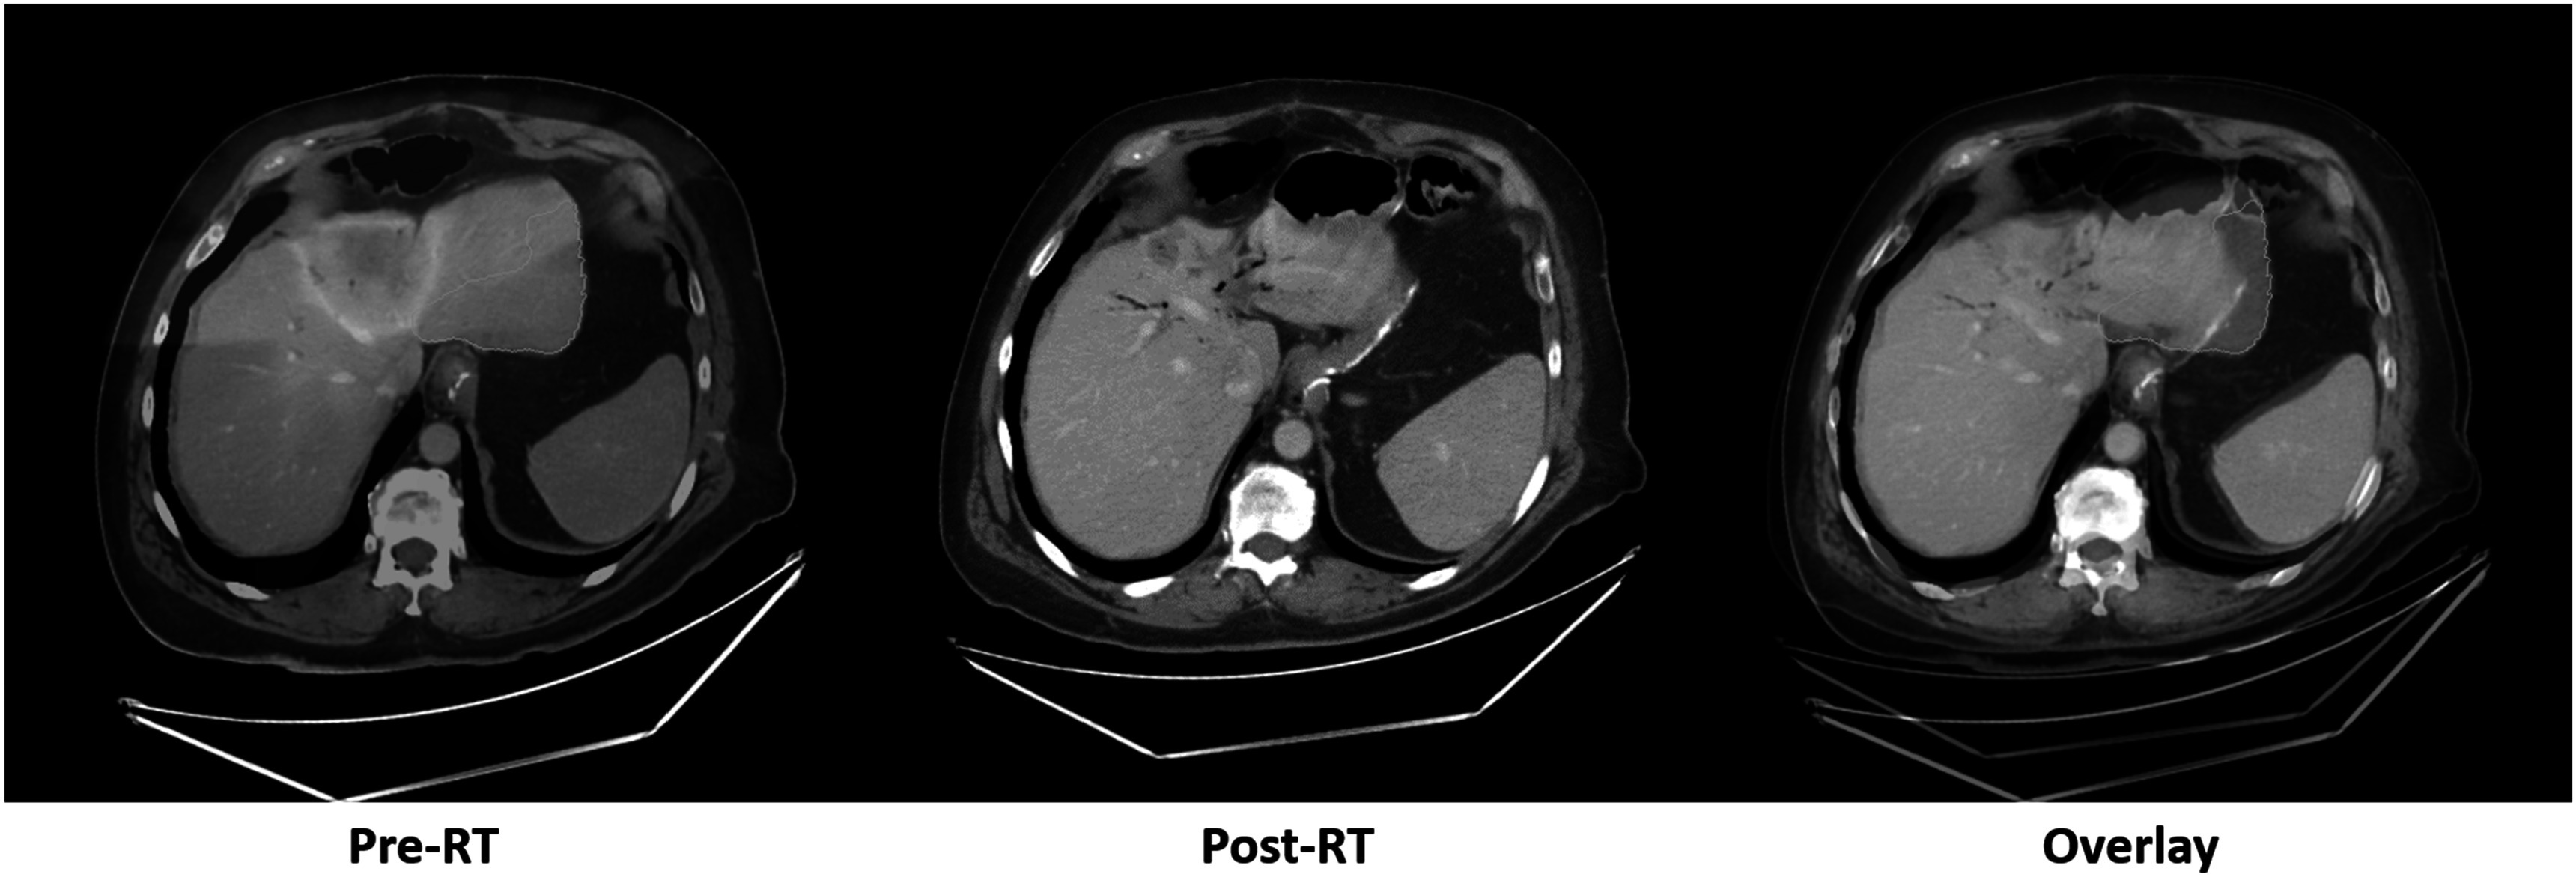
Example of an atypical case. Left: pre-RT CT scan with fused dose distribution and representation of segment 2 contour (cyan). Middle: post-RT CT scans exhibiting a clear shrinkage of the liver in the segment 2 region. Right: color overlay between the pre- and post-RT CT scans and representation of segment 2 (cyan).
